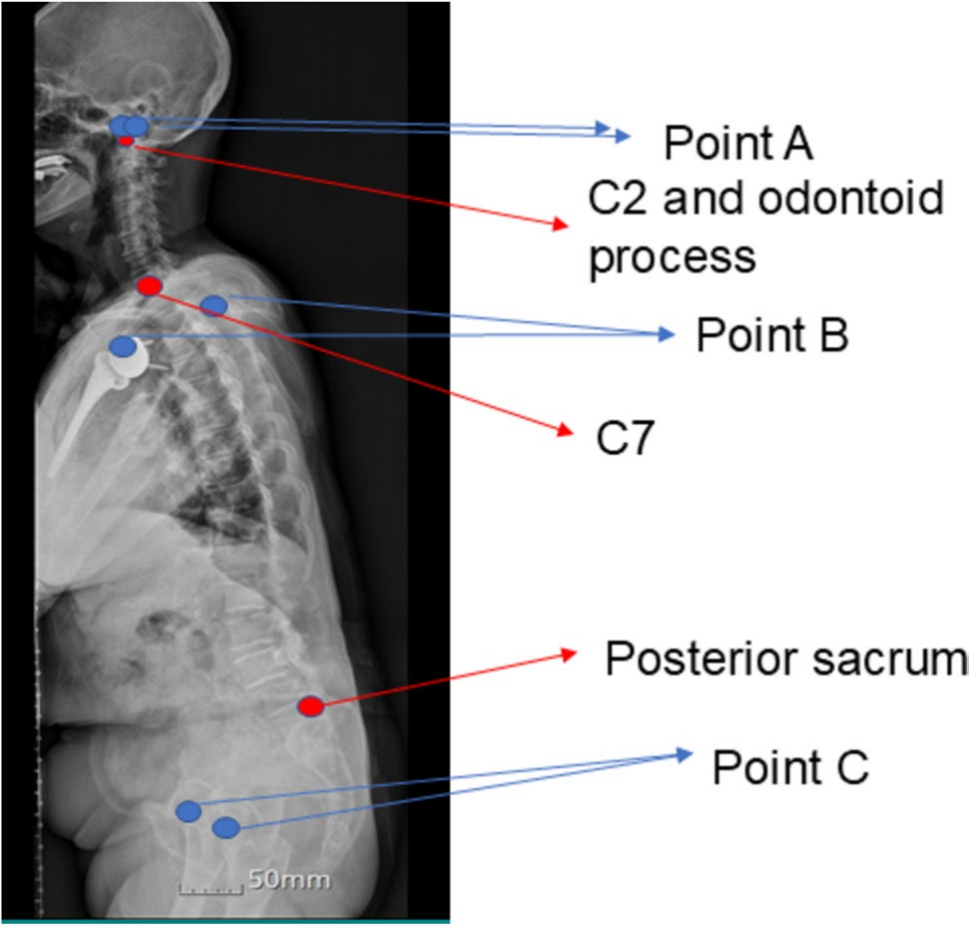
Point A,B,C – 3D scanning point / C2 and odontoid process, C7, posterior sacrum – x-ray point
